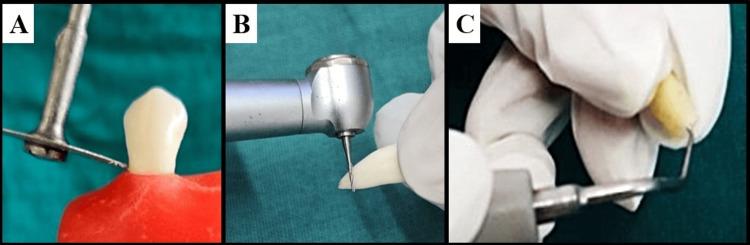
Tooth preparation A - Decoronating the obturated tooth to standardize the root length to 14 mm. B - 3 mm of root-end resection using a carbide fissure bur. C - Root-end cavity preparation using an ultrasonic tip
